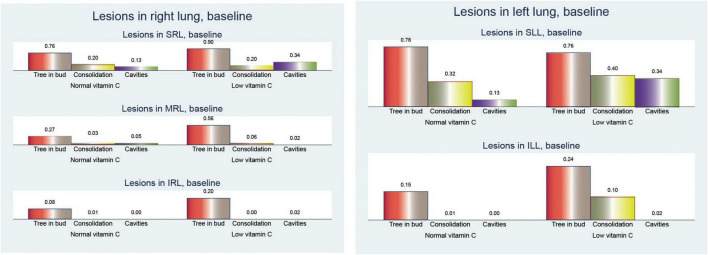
Pulmonary computed tomography (CT) lesions at baseline.
